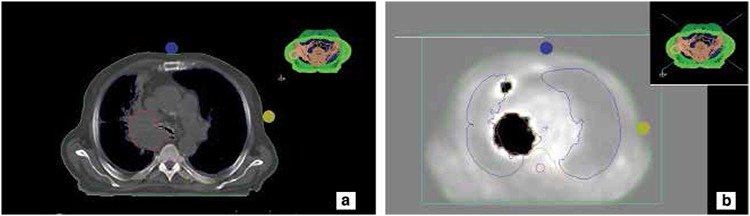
a) A normal-sized mediastinal lymph node in computed tomography images, b) Increased activity uptake was seen in positron emission tomography images, gross tumor volume included
